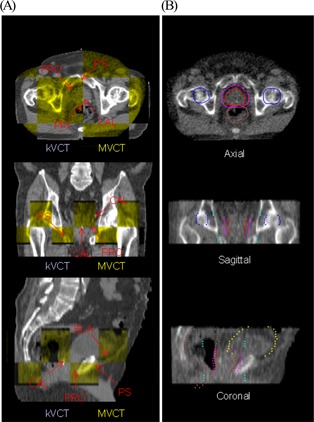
The image fusion tool on the TomoTherapy (Madison, WI) operation station is used to register the treatment planning kilovoltage computed tomography (kVCT) images with megavoltage computed tomography (MVCT) images. After an automated CT‐to‐CT fusion, the operator can manually adjust the registration (A) by using a “checkerboard” display or (B) by overlaying the treatment planning contours with the MVCT image. Axial, sagittal, and coronal views are shown for both registration techniques. Arrows in the “checkerboard” display point to anatomic landmarks used in manual adjustments for prostate patients. The prostate (red), planning target volume (pink), rectum (brown), bladder (yellow), and femoral heads (blue) are shown in the contour overlay. PRO=prostate; PS=pubis symphysis; CAL=calcification; PRI=prostate−rectal; BLA=bladder.
